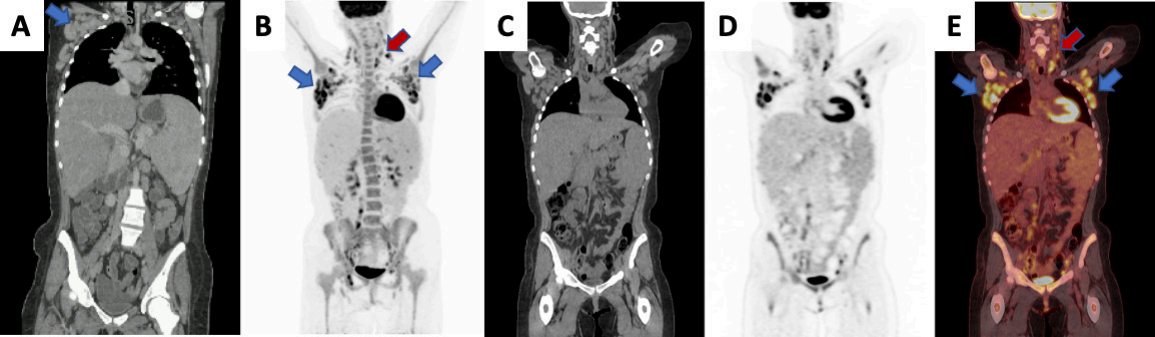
Initial CT (A) demonstrates lymphadenopathy above and below the diaphragm. Half body [18F]FDG PET/CT scan: Anterior Maximum Intensity Projection (MIP) (B) shows avid bilateral cervical/ supraclavicular (red arrows), axillary (blue arrows), retroperitoneal and iliac chains. Coronal images (C-E) demonstrate hepatosplenomegaly with elevated splenic uptake in relation to that of the liver (liver SUV max 2.2 and spleen SUV max 3.2) and diffuse increased marrow uptake. There is no abnormal cutaneous or periarticular uptake.
